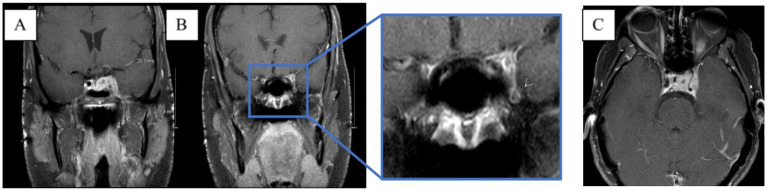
MRI brain T1 + C when the patient lost corneal and pupillary reflexes. Subsequently transferred to the ICU. (A) Patchy enhancement within basal ganglia. (B) Worsened leptomeningeal enhancement around the suprasellar cistern. (C) Non-enhancing cystic focus suggesting thrombus or abscess.
